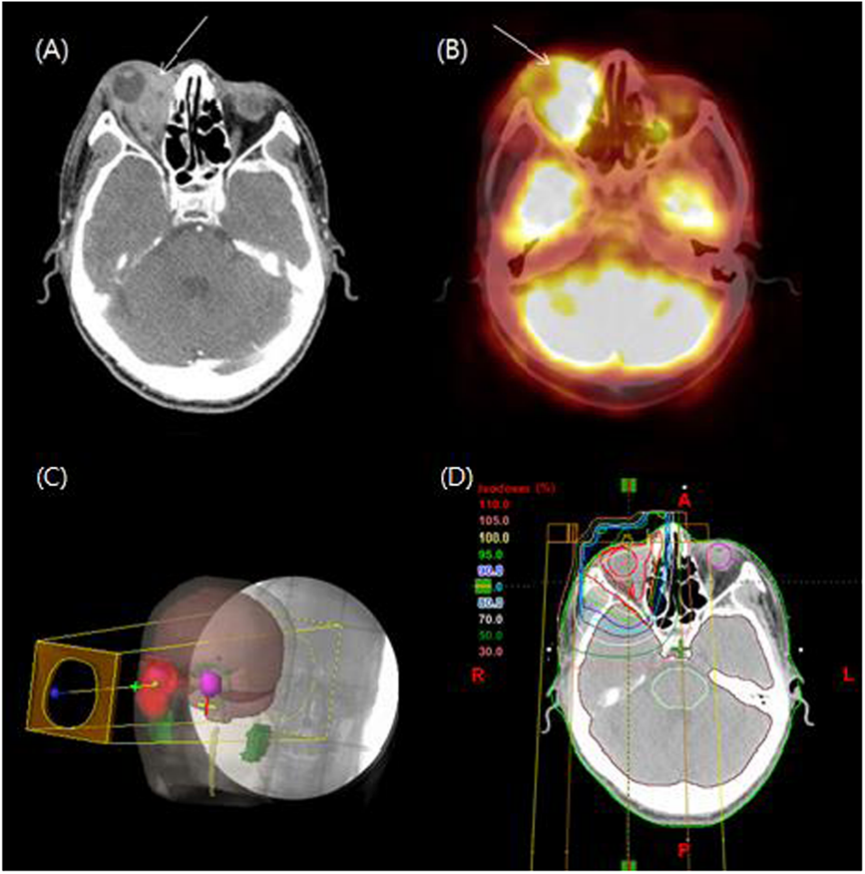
Compared to CT and PET scan 3 months ago. (A) Follow-up CT scan shows developed homogenous enhancing lesion surrounding the right orbit and right lower eyelid, and (B) the hypermetabolic lesion in the right orbit were newly disappeared in the follow-up PET scan. (C, D) The patient received RT with high energy electron to the right orbit.
